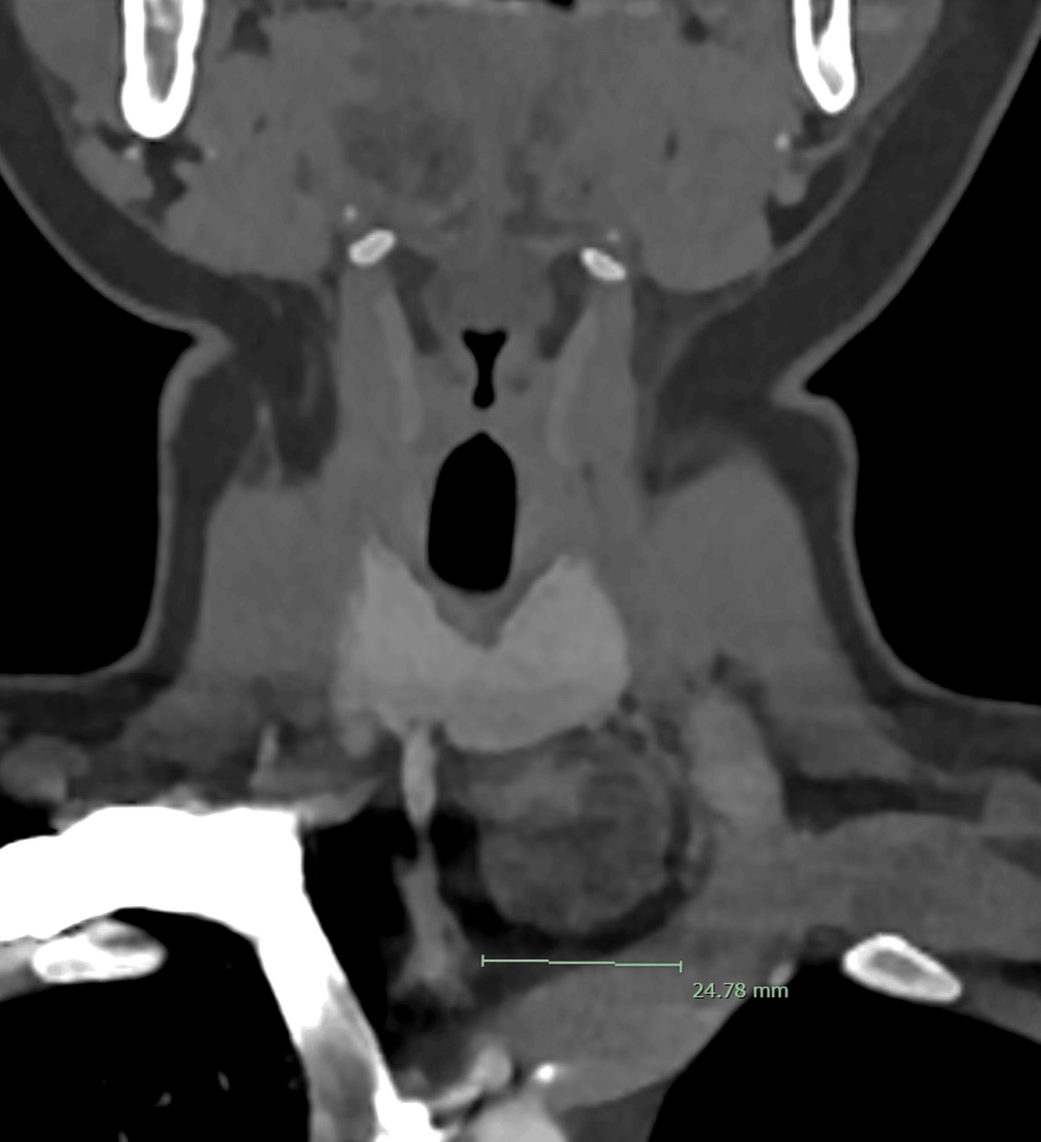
4D-CT scan: coronal view
